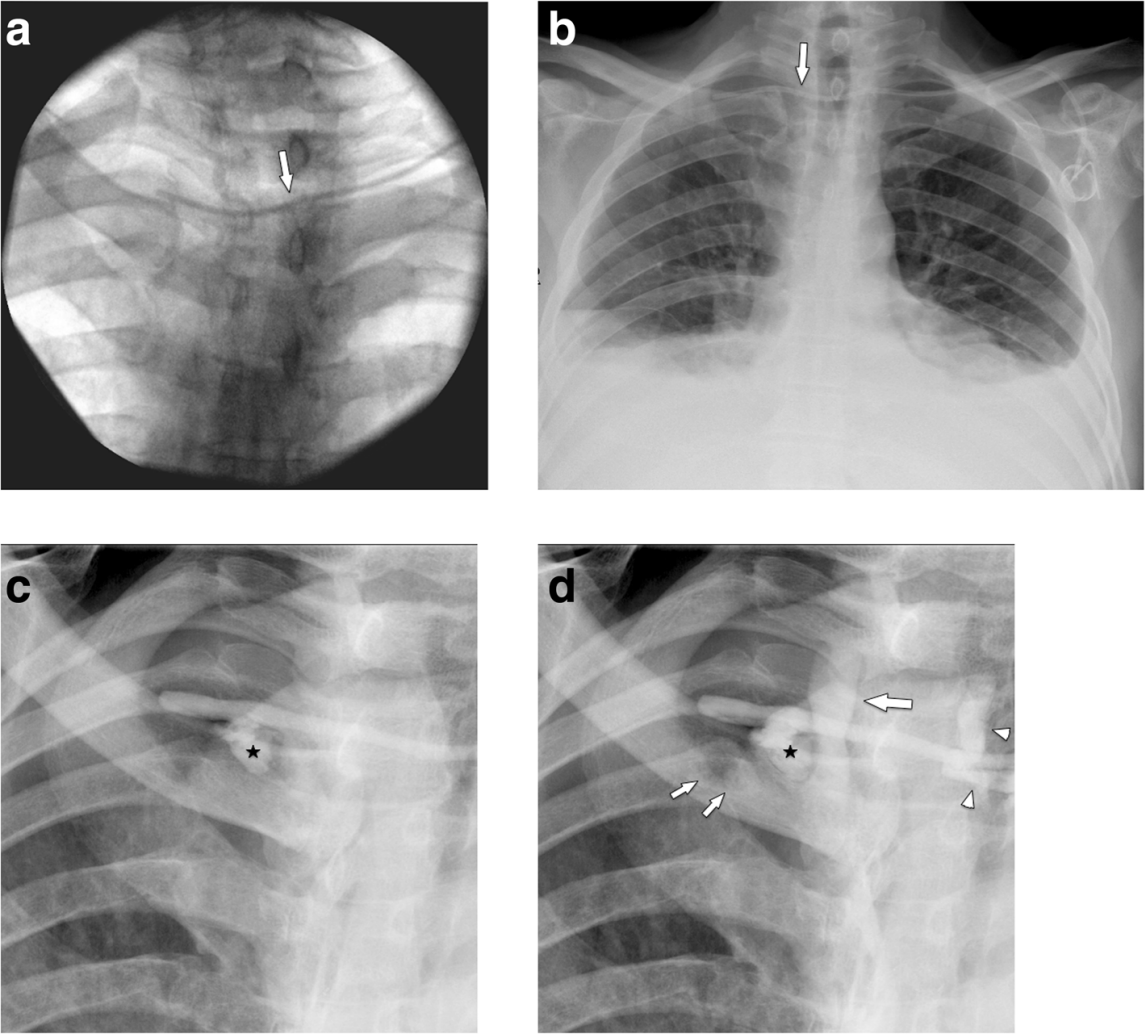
Extravascular malpositioning. a Intraoperative fluoroscopy shows the catheter projecting above the clavicles, which is not in line with an intravascular position (in the brachiocephalic vein). b Port system was subsequently used for several weeks. Follow-up chest radiograph shows increasing pleural effusions. Malposition of the catheter was reported. c, d Subsequently a flow confirmation study was performed, which shows extravascular collection of contrast (star) and some contrast material reflux into the internal jugular vein (large arrow), the subclavian vein (small arrow), as well as along the catheter into cervical veins (arrowhead). The catheter has an extravascular course and perforated the contralateral venous angle, with chemotherapy infusions partly entering the venous systems, partly filling up the pleural space
